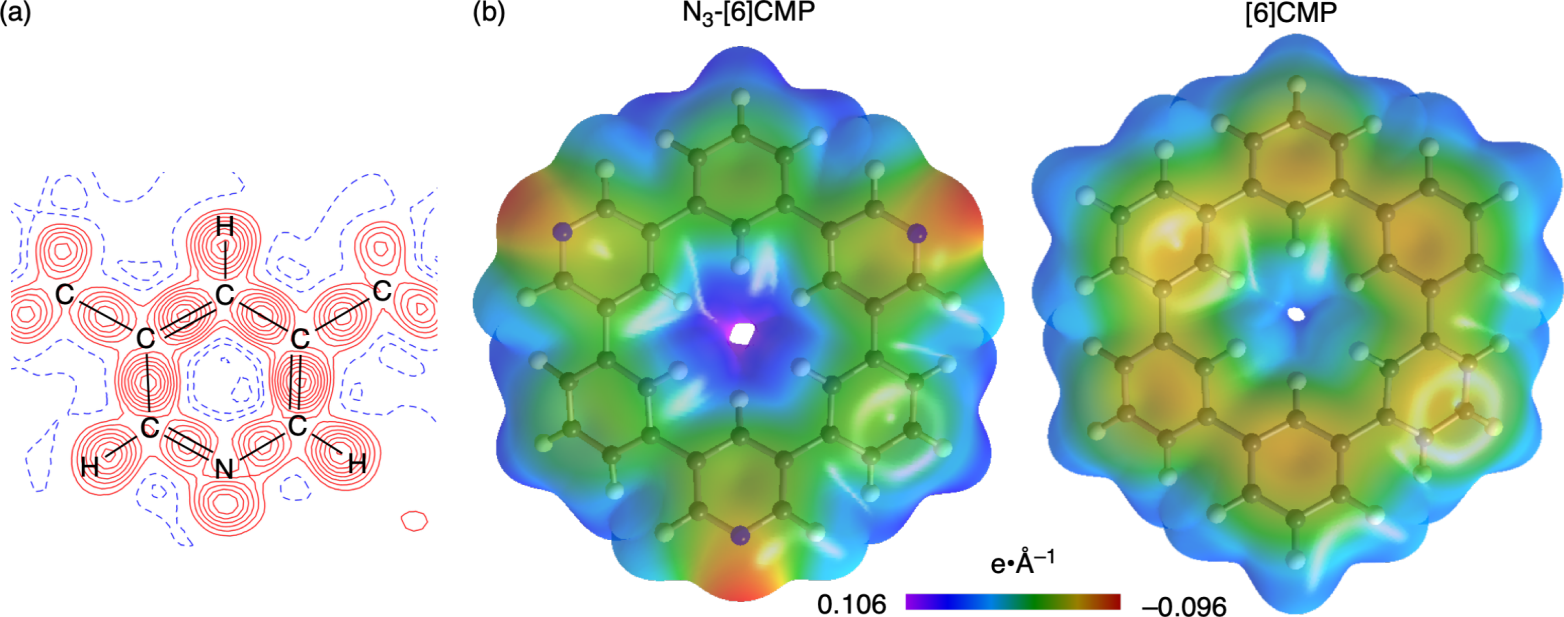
X-ray charge density analyses of 3a and [6]CMP. (a) Deformation map (*F*_o_ – *F*_c_) of a pyridine ring in 3a (contour interval: 0.05 e·Å^−3^, positive: red, negative: blue). (b) Electrostatic potential maps mapped on the 0.0067 e·Å^−3^ isosurface for the electron density.
